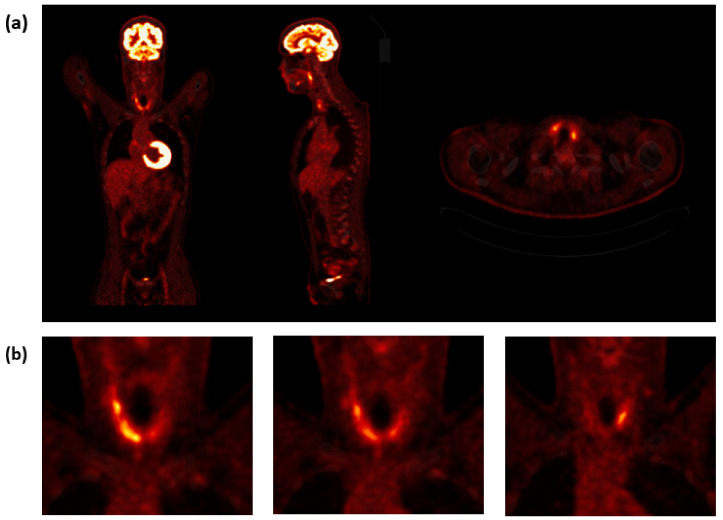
2-[^18^F]FDG PET/CT imaging. (a) Coronal, sagittal, and transversal 2-[^18^F]FDG PET/CT images slices showing high tracer uptake in both lobes of the thyroid. (b) Representative coronal PET/CT images of multiple 2-[^18^F]FDG-hot spots in both thyroid lobes, mostly on the right. PET/CT, positron emission tomography/computed tomography; 2-[^18^F]FDG, 2-deoxy-2-[^18^F]fluoro-d-glucose.
